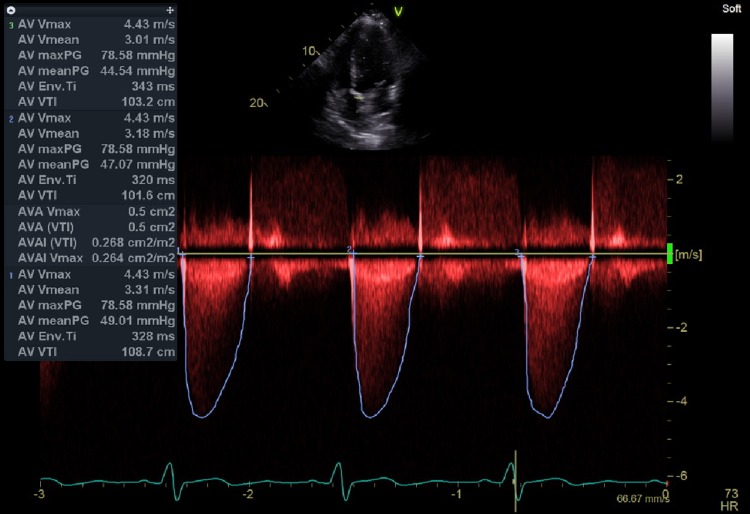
Transthoracic Echocardiogram showing Aortic Valve Area, Peak Velocity and Mean Gradient Consistent with Severe Aortic Stenosis
